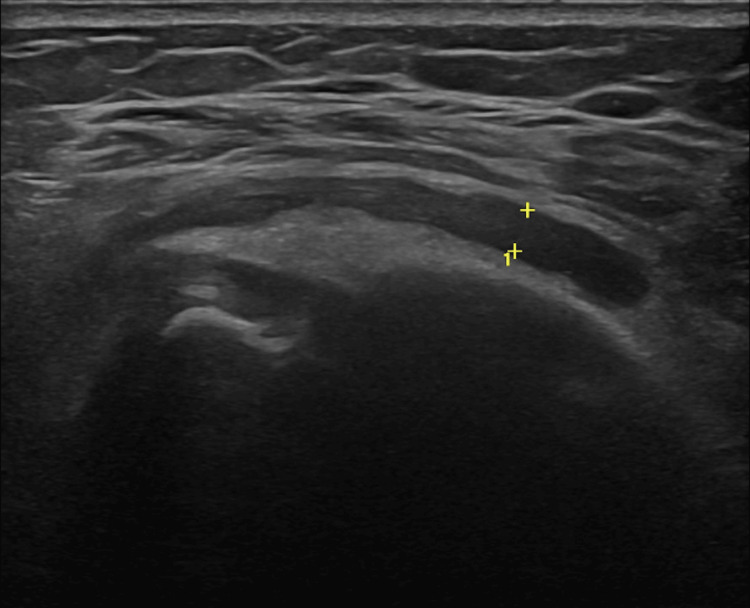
Left shoulder ultrasound Small joint effusion is observed with a maximum thickness of 4 mm, accompanied by some thin and sparse internal septa, suggesting inflammatory involvement.
